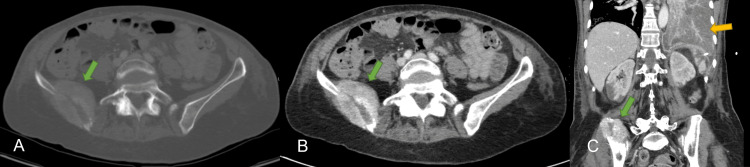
CT of the pelvis showing a right iliac bone mass with a soft tissue component (green arrows), highly suggestive of a metastatic lesion Note the consolidation of the entire left lower lobe (yellow arrow).
